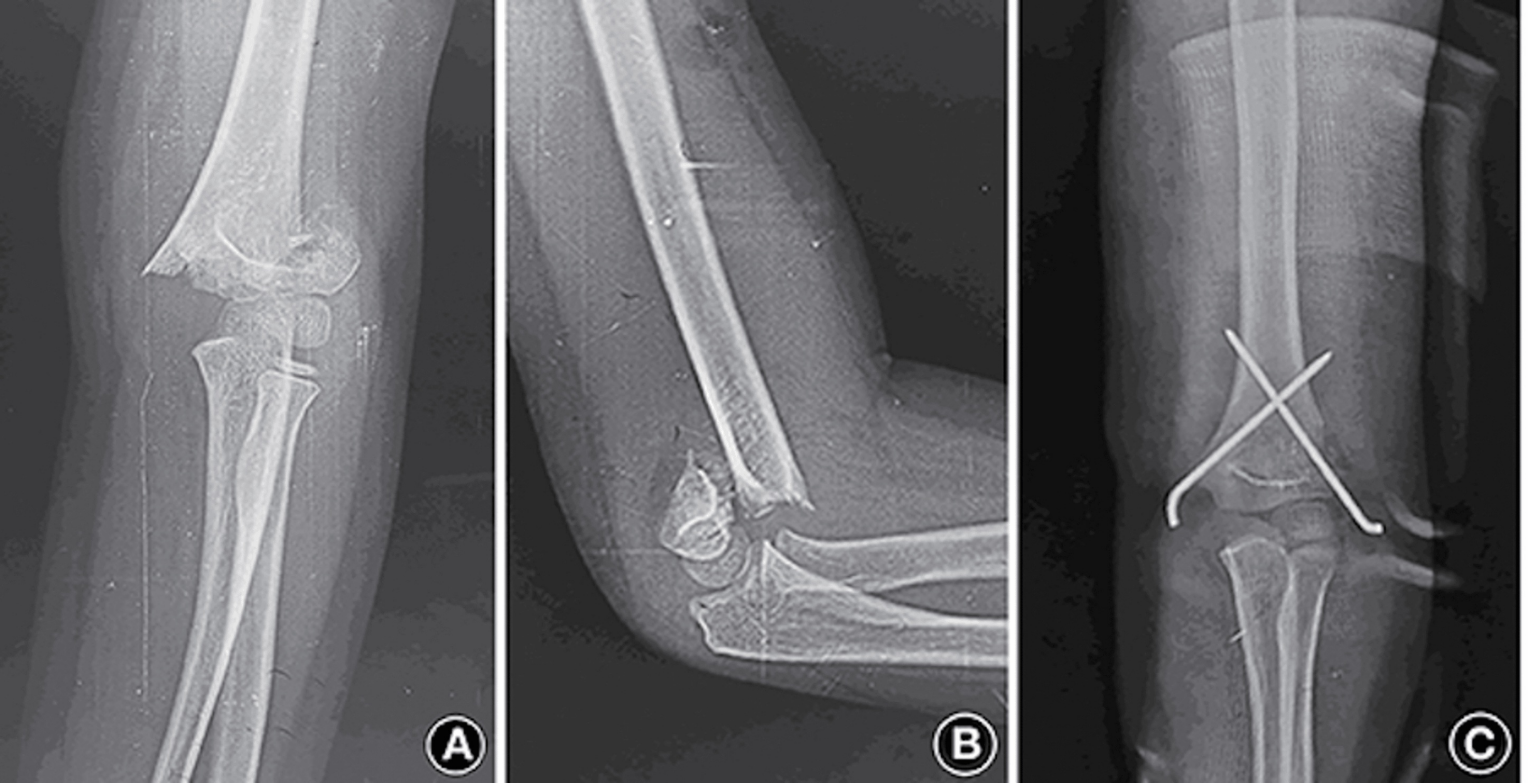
Type 3 SCFH treated with cross K-wire fixation A and B: Preoperative anteroposterior and lateral radiographs of an eight-year-old boy; C: Immediate postoperative radiograph with good reduction SCFH: Supracondylar fracture of the humerus, K-wire: Kirschner wire
